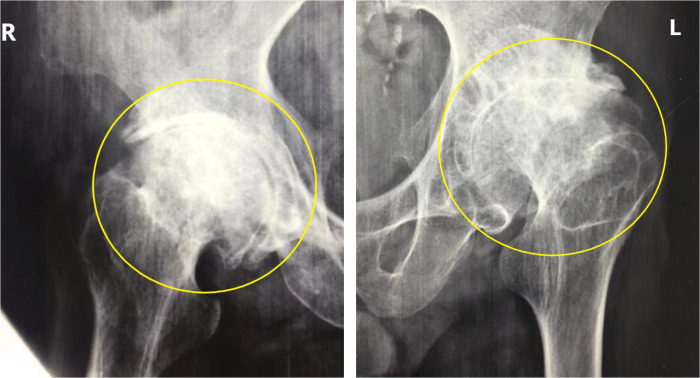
The patient's preoperative x-ray of the hip joint. (Figure notes: Circles in the x-ray were marked to show that varying degrees of necrosis of both femoral heads, with a large amount of osteophytes forming around the hip joint).
